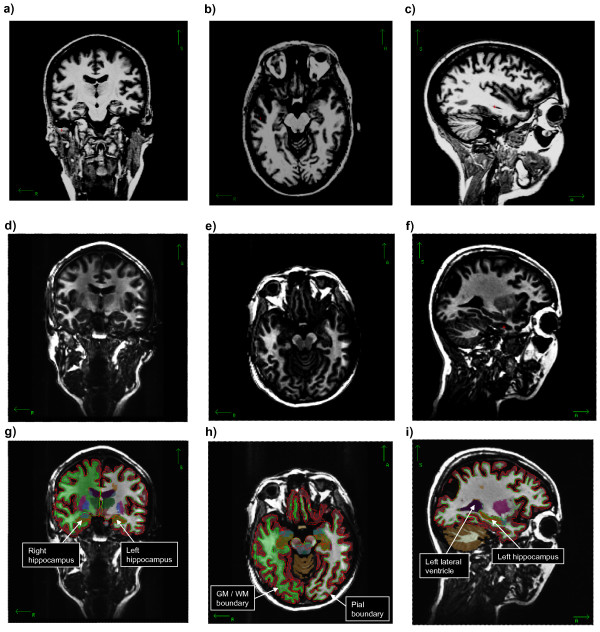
Original MRI data (upper two rows) and color-coded segmented data (lower row). a) Coronal slice 120 from an Oslo dataset. b) Axial slice 120. c) Sagittal slice 160 transectioning left hemisphere. d) Coronal slice 120 from a Bergen dataset. e) Axial slice 112. f) Sagittal slice 160 transectioning left hemisphere. g) Same slice as in d) with pial boundary, gray matter/white matter boundary, and subcortical segmentation, with annotation of segmented left and right hippocampus. h) Same slice as in e) with overlayed segmentations and annotation of pial surface and gray matter/white matter boundary. i) Same slice as in f) with overlayed segmentations and annotation of segmented left lateral ventricle and left hippocampus.
